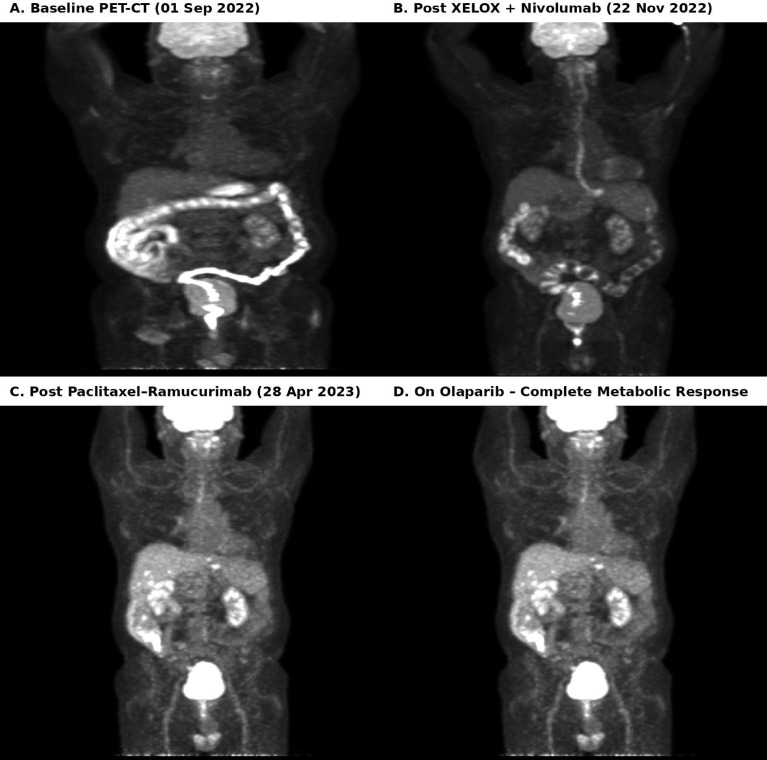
Serial FDG PET-CT images demonstrating disease progression after chemo-immunotherapy and subsequent complete metabolic response following olaparib treatment.
